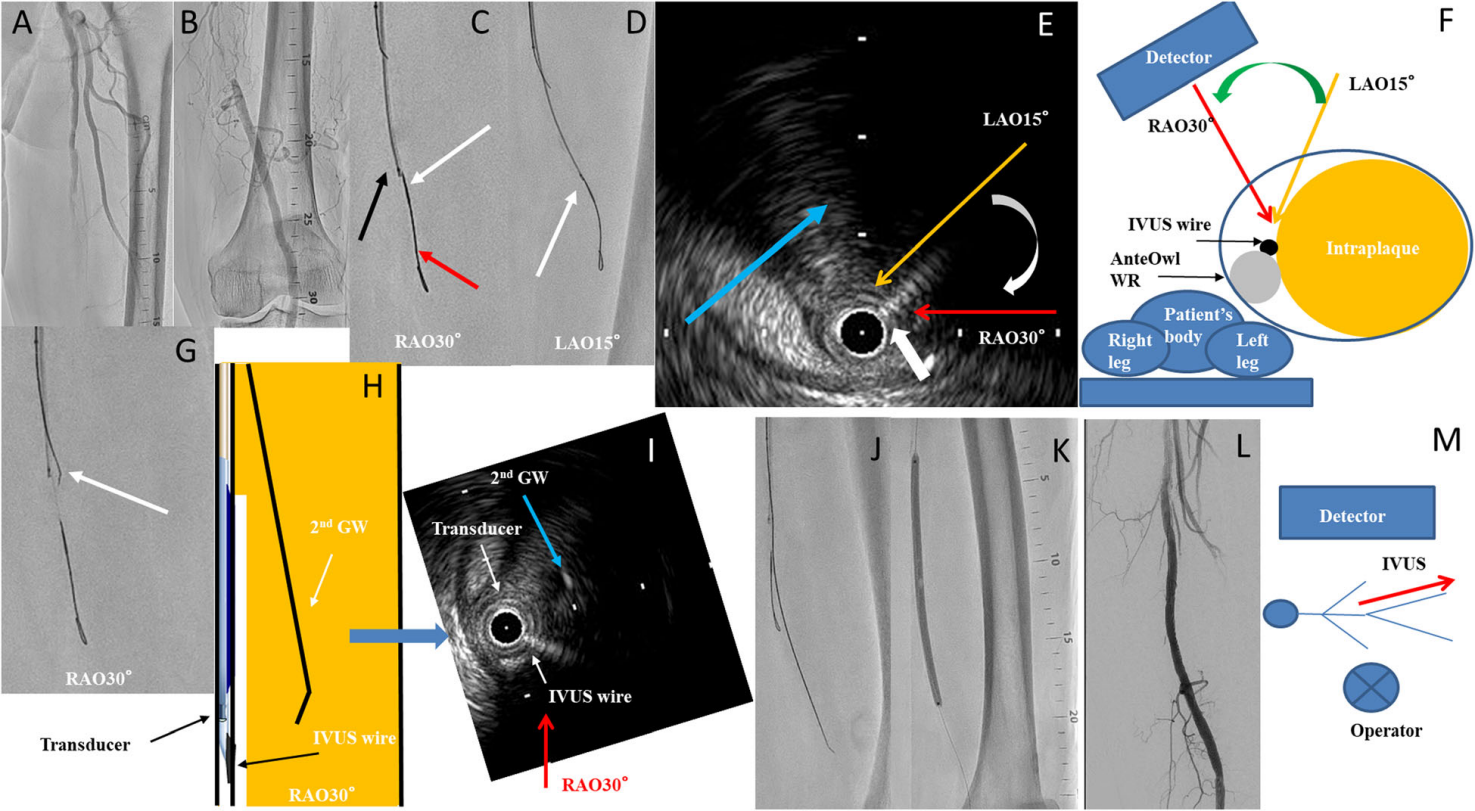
a, b Control angiography showed a tandem stenotic lesion in the left proximal superficial femoral artery and total occlusion of the left middle to distal aspects of the superficial femoral artery. c A 0.014-in. guidewire was advanced into the subintimal space (red arrow shows the tip of the guidewire). The black arrow shows the intravascular ultrasound (IVUS) transducer, and the white arrow shows the IVUS wire. The transducer was on the left side of the IVUS wire. d The transducer almost overlapped the IVUS wire (white arrow). On the basis of these findings, we confirmed that the IVUS transducer was at the bottom and the IVUS wire was at the top. e IVUS findings of the chronic total occlusion lesion. The white arrow shows the IVUS wire. The detector direction where the transducer and IVUS wire coincided on the angiographic image was left anterior oblique (LAO) 15° (yellow arrow). The detector direction where the transducer and target plaque were maximally separated at right anterior oblique (RAO) 30° (red arrow) was at 45° rotation to a clockwise direction from LAO 15° to RAO 30° (white arc arrow). The center of the target plaque was a few millimeters to the right of IVUS (blue arrow). f Cross-sectional image from the distal position (operator’s position). The IVUS wire and transducer coincided with LAO 15° and RAO 30° was maximally separated of IVUS and target plaque. The IVUS wire was on the surface side of the transducer, and the target plaque was on the right side of the IVUS catheter. g The second guidewire was advanced a few millimeters to the right of IVUS on the angiography (white arrow). h, i By rotating RAO 30° on the IVUS image to 6 o’clock according to the direction of guidewire advancement, the angiographic image and the IVUS image were visually matched. The IVUS catheter was in the subintimal space. The second guidewire was advanced a few millimeters to the right of the IVUS catheter on angiography, and the right blue arrow shows that the second guidewire could be advanced almost into the center of the target intraplaque space in the IVUS image. j The second guidewire could be passed through the chronic total occlusion lesion. k, l We dilated the drug-coated balloon. Final angiography showed a good outcome. m Positional relationship between patient and operator and direction of IVUS
